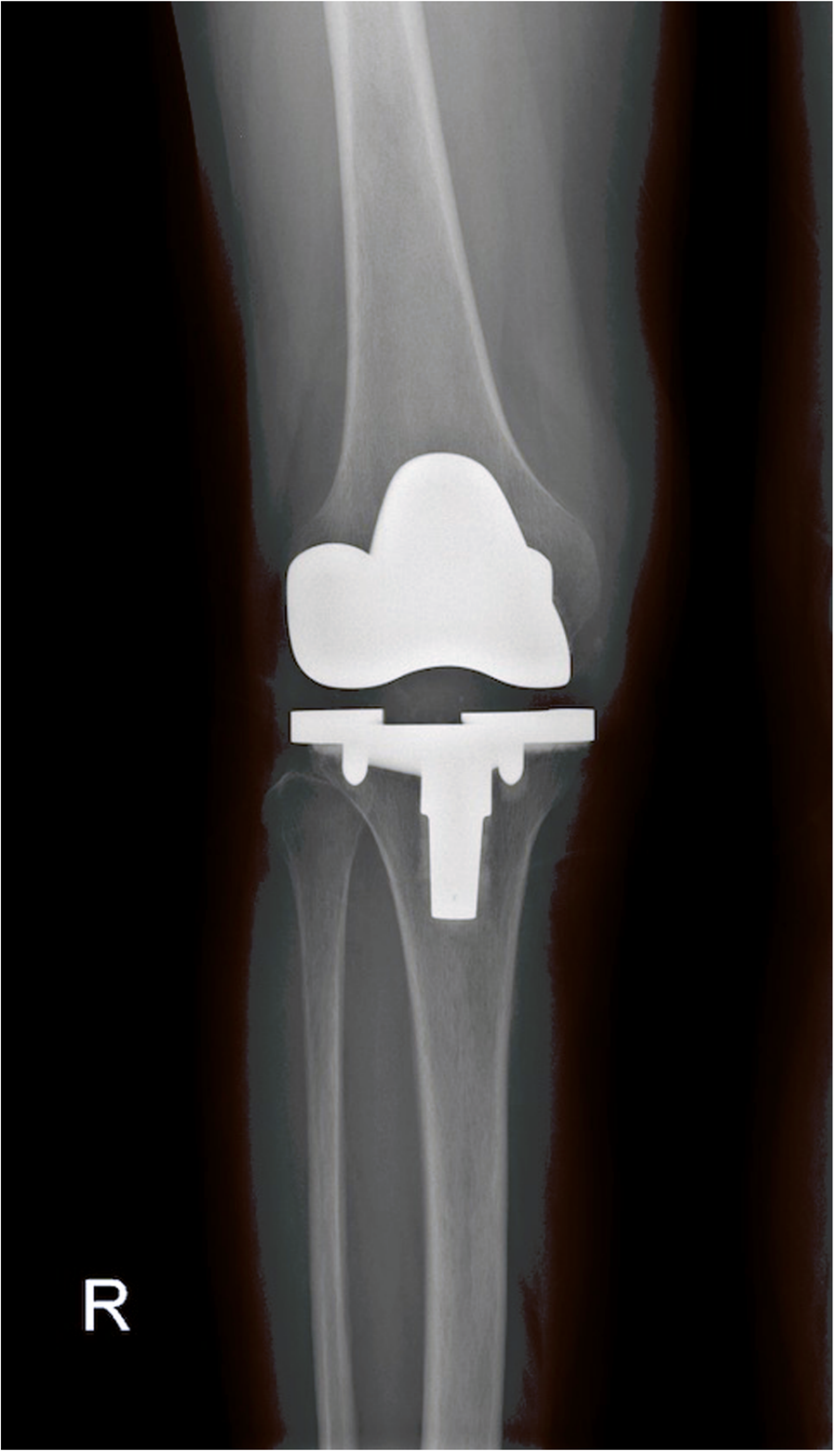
A radiograph of PVNS was taken 6.2 years after TKA showed well-fixed prosthesis
